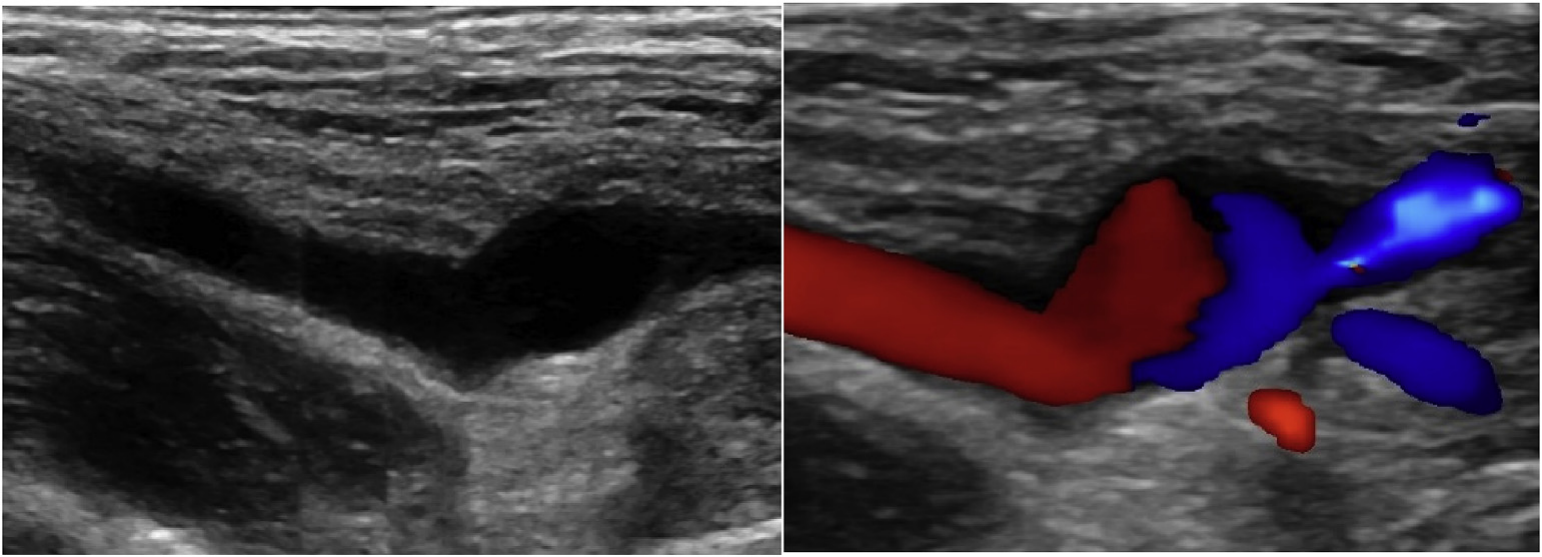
Ultrasound and Doppler images of the left upper extremity which shows fusiform aneurysmal dilatation of the brachial artery with turbulent flow.
